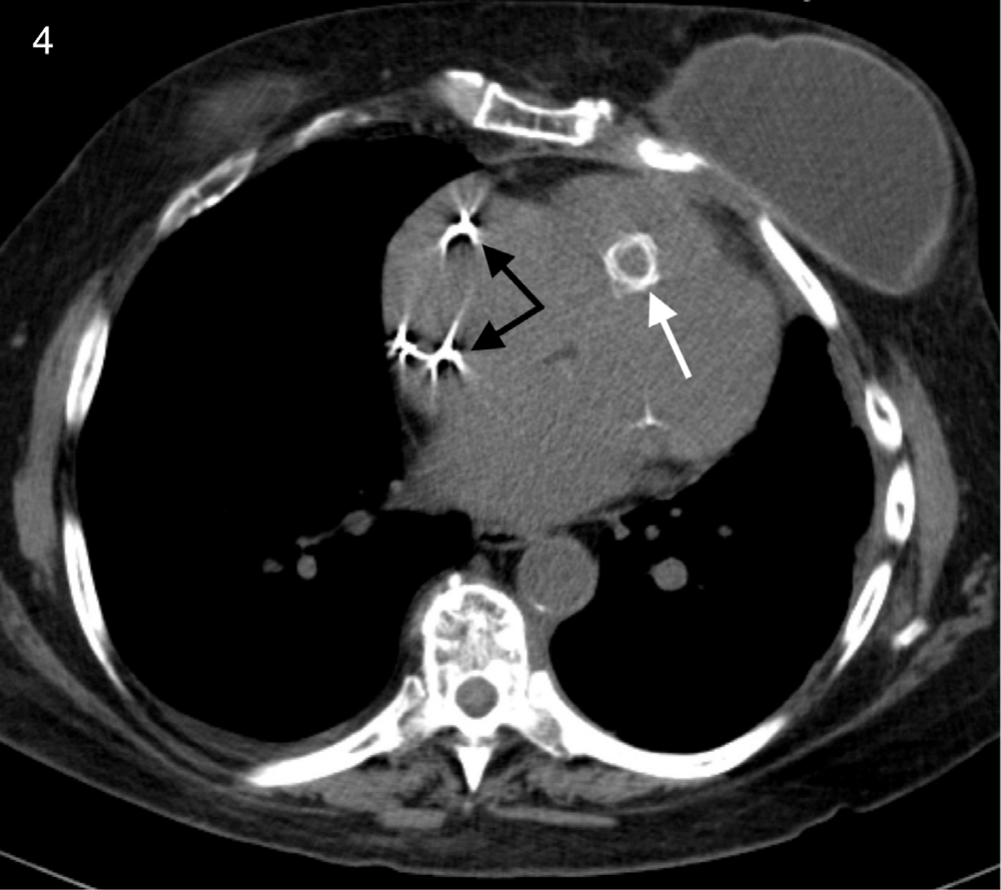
Axial view CT demonstrating a migrated stent in the right ventricular outflow tract (white arrow). Black arrows indicate pacemaker leads placed for complete heart block.
